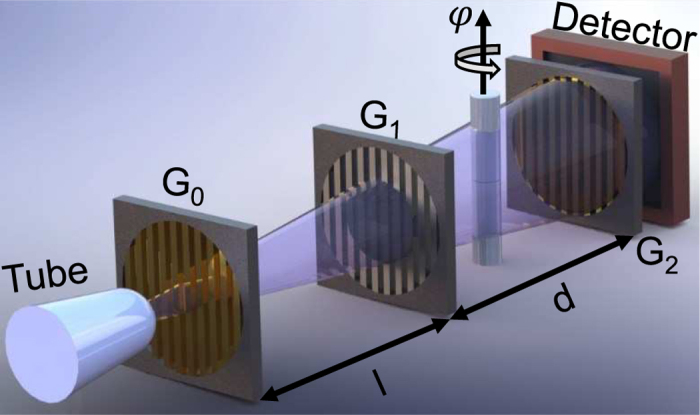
The experimental setup consisting of (from left to right) the X-ray tube, the source grating *G*_0_, the phase grating *G*_1_, the sample (cylinder) and the tomographic rotation axis (indicated by the black arrow pointing upwards), the analyzer grating *G*_2_ and the X-ray detector. The inter grating distances *l* and *d* were both 92.5 cm. Further details on the setup can be found in ref. 12. During a CT scan the sample is rotated around the tomographic axis while images are acquired for several angles *φ*.
